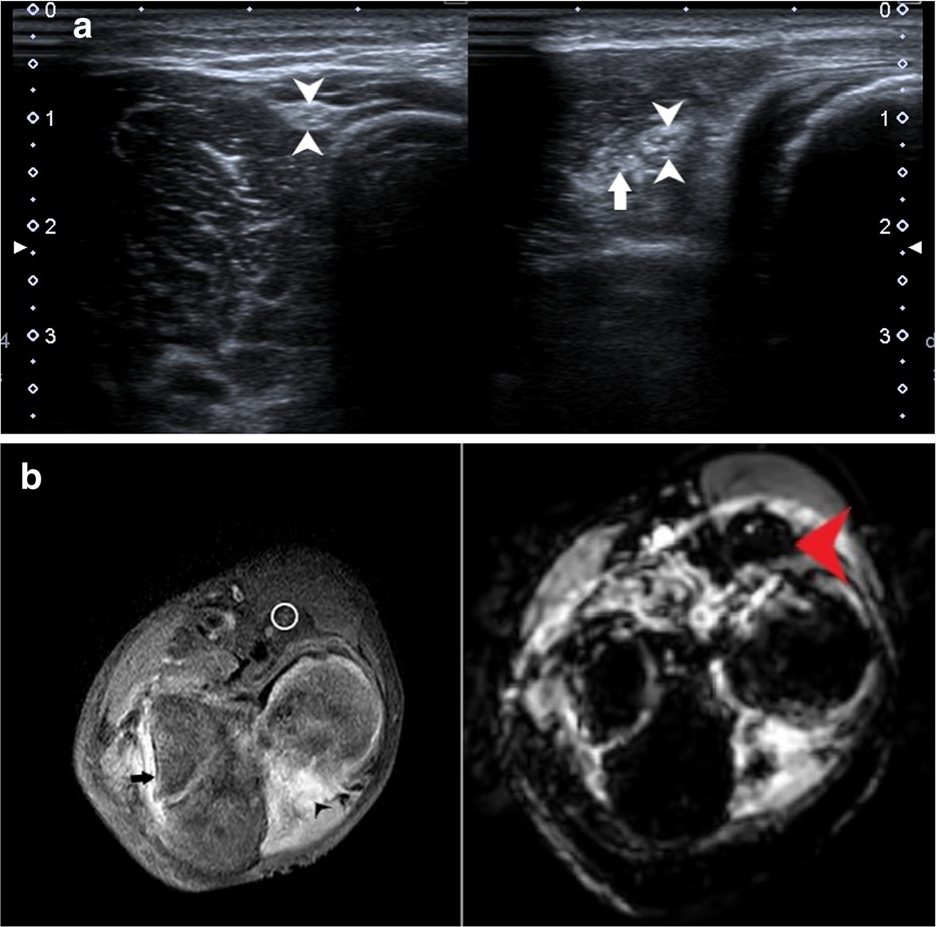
a Normal radial nerve (between white arrowheads) at the level of the spiral groove on the left with a mildly hypoechoic radial nerve surrounded by echogenic soft tissue (white arrow) in the distal arm as seen in the panel on the right. Based on ultrasound findings, radial nerve entrapment in scar tissue or hematoma was suspected. b The radial nerve (encircled), just before its division into the superficial and deep components is hyperintense on PDFS image with surrounding hypointense soft tissue. There are also changes of haemophilic arthropathy as evident by the hyperintense joint effusion (arrowheads), cortical irregularities and cartilage thinning (black arrow). On corresponding SWI image, there is blooming in the area around the radial nerve, which corresponds to the echogenic soft tissue on ultrasound (red arrowhead). This was a case for radial neuropathy due to entrapment in local hematoma in a case of haemophilia
